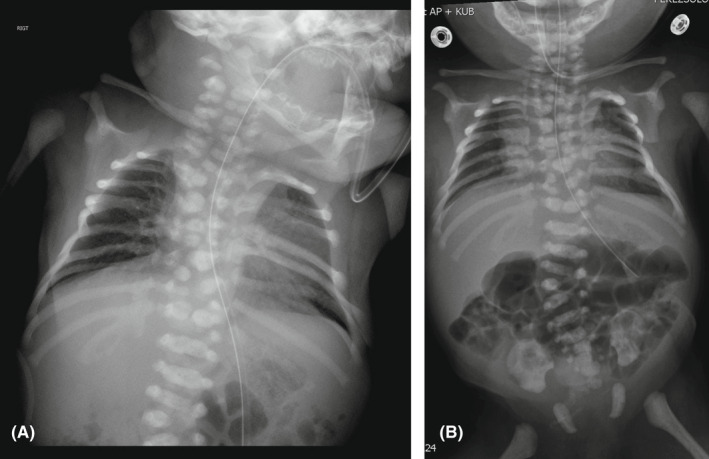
(A and B) Spinal radiographs shows segmentation anomalies of thoracic spines and rib abnormalities, L3 and L4 hemivertebra with lumbar scoliosis
